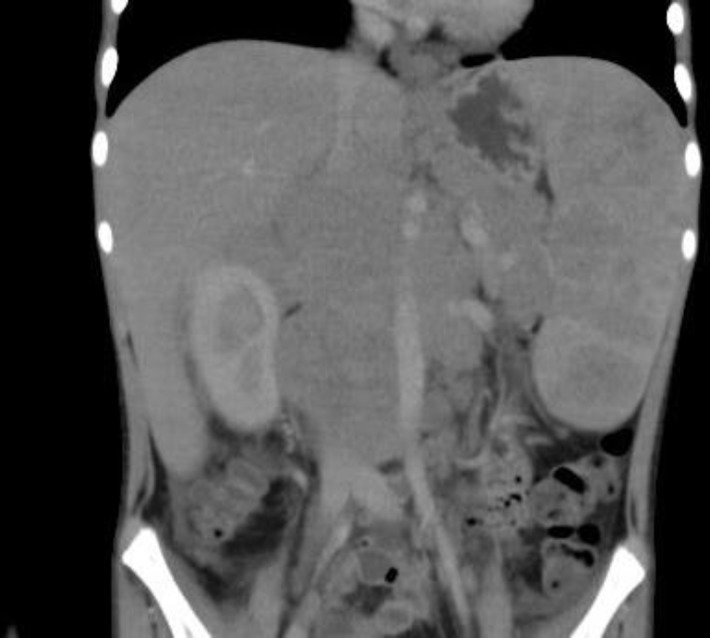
Contrast enhanced Computed Tomography (CECT) abdomen showing homogenously enhancing conglomerated mass forming lymph nodes in aortic and para-aortic region with encasement of abdominal aorta, IVC, and renal vessels extending from D11 to L4 vertebrae.
